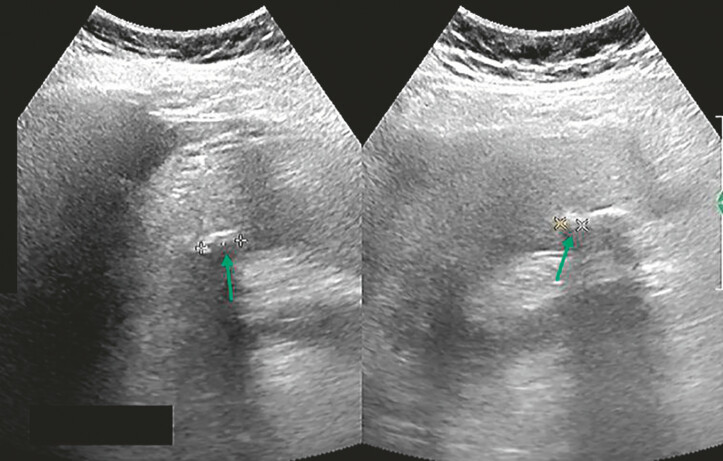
Abdominal ultrasound reveals multiple gallstones with cholecystitis in an 84-year-old female patient, measuring 0.8–1.2 cm in diameter. Arrows indicate the location of the stones.
